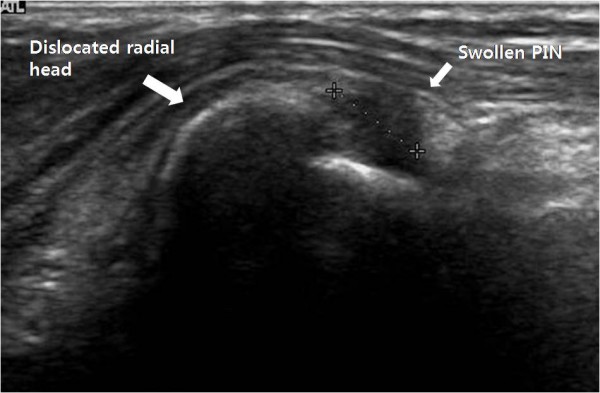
Ultrasound image showing compression of the posterior interosseous nerve by the dislocated radial head, with focal swelling.
